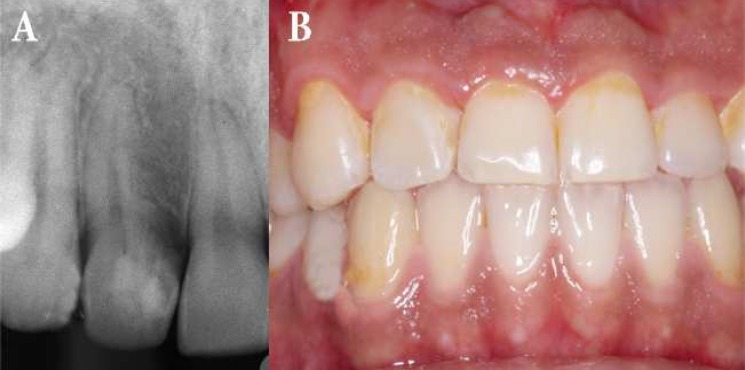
Seven-years after completion of surgical/orthodontic treatment. *A)* Periapical radiography showing complete bone healing. The pulp of the asymptomatic tooth continued to provide positive responses to pulp-sensibility testing and periodontal probing depths were within normal limits, *B)* Facial clinical appearance of the case
